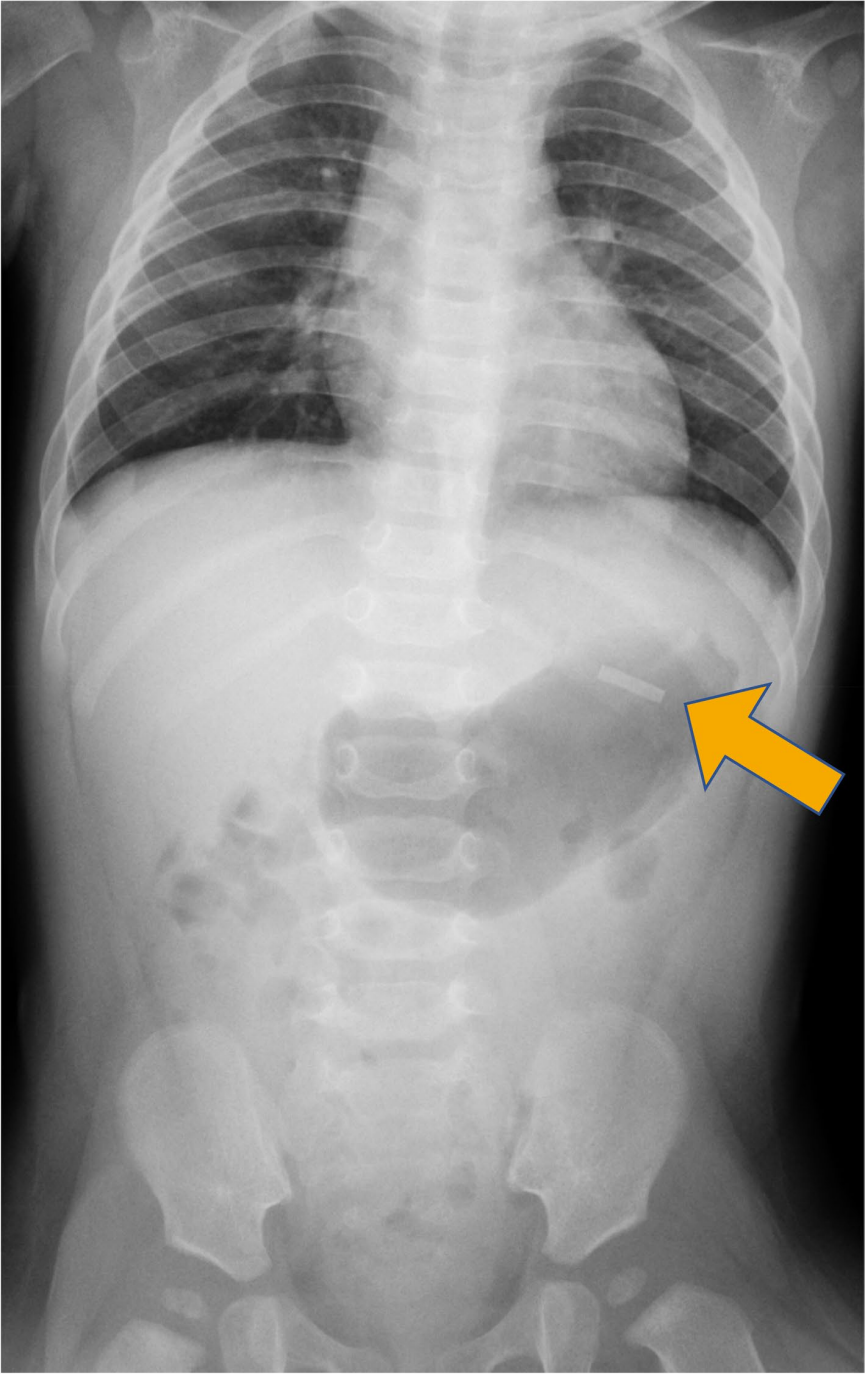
Initial abdominal X-ray. The X-ray revealed a metallic foreign body in the stomach (arrow)
